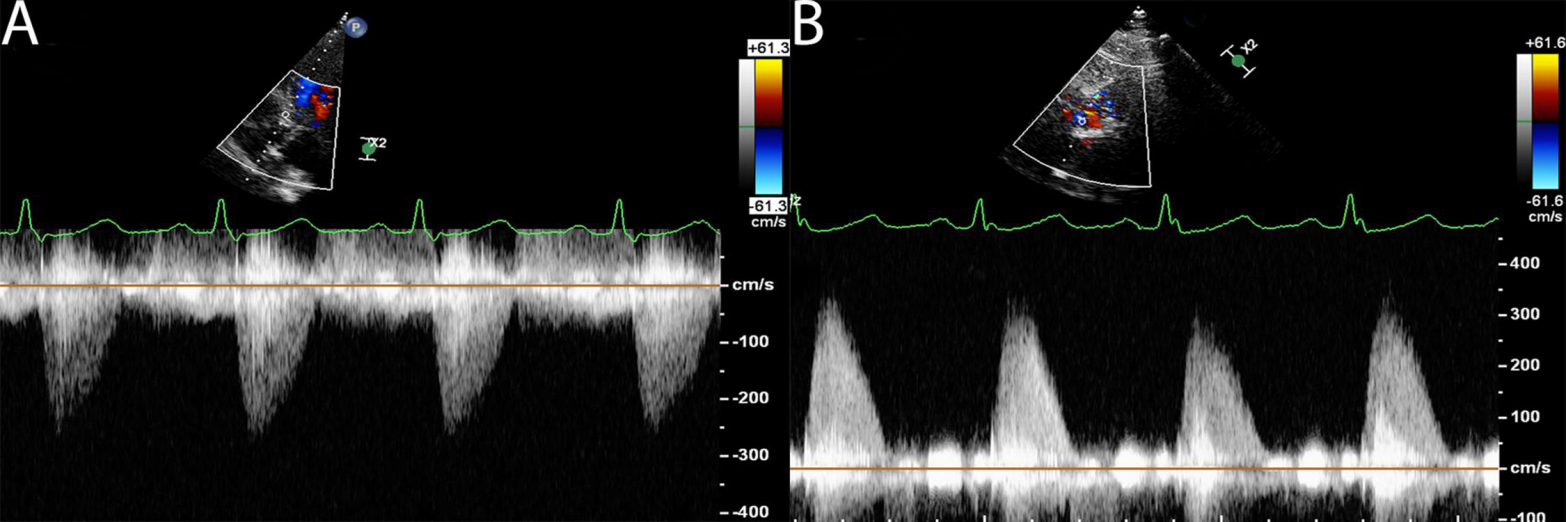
Apical 5-chamber (AP5Ch—(A)) and right sternal edge (RSE—(B)) continuous wave spectral Doppler tracings from a patient with aortic stenosis. Left ventricular outflow tract (LVOT) diameter was 2.1 cm and LVOT velocity time integral (VTI) 22 cm. Peak transvalvular velocity, mean gradient and effective orifice area were 2.6 m/s, 13 mm Hg and 1.40 cm^2^, respectively from the AP5Ch view but 3.4 m/s, 21 mm Hg and 1.05 cm^2^, respectively using RSE Doppler data. Thus, *discordant* moderate aortic stenosis (AS) became *concordant* moderate AS using the non-apical continuous wave Doppler data.
